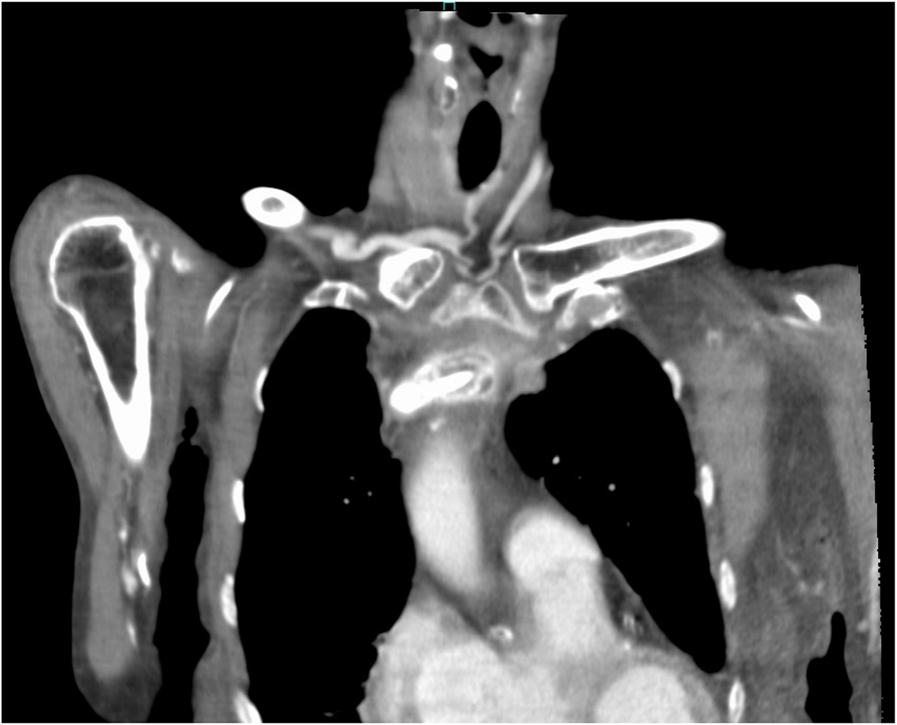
Chest computed tomography scan (coronal)
